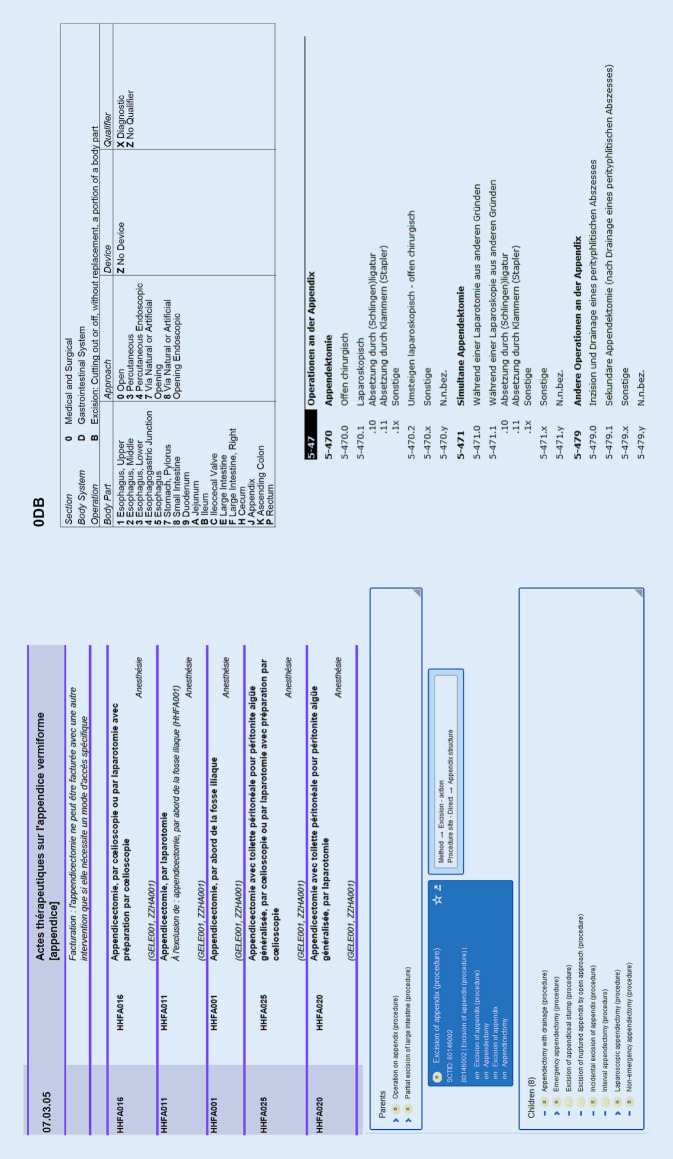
Was bedeutet das für den grenzübergreifenden Datenaustausch? Wenn Menschen reisen und an verschiedenen Orten eine medizinische Behandlung in Anspruch nehmen, so wäre ein Zugriff auf die versorgungsrelevanten Daten, wie z. B. Allergien, Implantate, aber auch vorangegangene Operationen oder dergleichen, hilfreich. Hierfür wurden entsprechende Strukturen auf europäischer Ebene geschaffen, die europäischen grenzübergreifenden Gesundheitsdienste MyHealth@EU^12^. Zur Nutzung dieser Strukturen ist auch eine Einigung auf europäische semantische und syntaktische Standardisierungen erforderlich. Für chirurgische Eingriffe hat man sich auf das Kodiersystem SNOMED CT geeinigt, allerdings bisher noch begrenzt auf die wichtigsten Kodes, die z. B. bei einer Notfallversorgung relevant sein könnten (Abb. 2).
